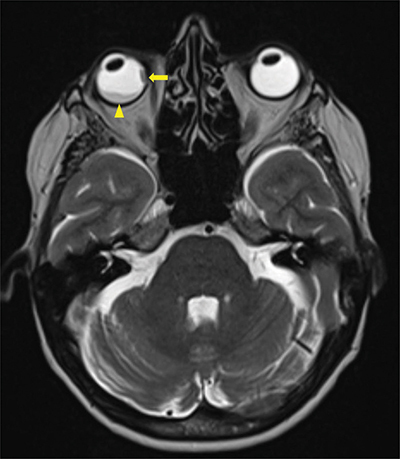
Axial T2 Dixon MRI-scan showing a second intra-orbital metastasis (arrow) invading all layers of the eyeball including sclera and a secondary retinal detachment (arrowhead).
